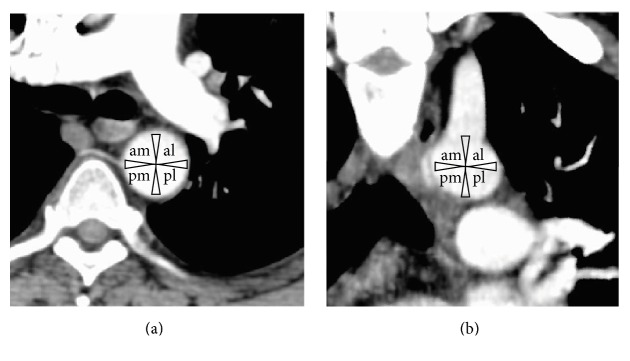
Ostium location methodology for bronchial arteries. (a) is cross-section of a thoracic CT scan when the origin is from the thoracic aorta, and (b) is a coronal section of a thoracic CT scan when the origin is from the aortic arch. Assessment of the ostium location of each bronchial artery at its origin is completed using the methodology described by Battal et al. [23]. am = anterior-medial, al = anterior-lateral, pm = posterior-medial, and pl = posterior-lateral.
